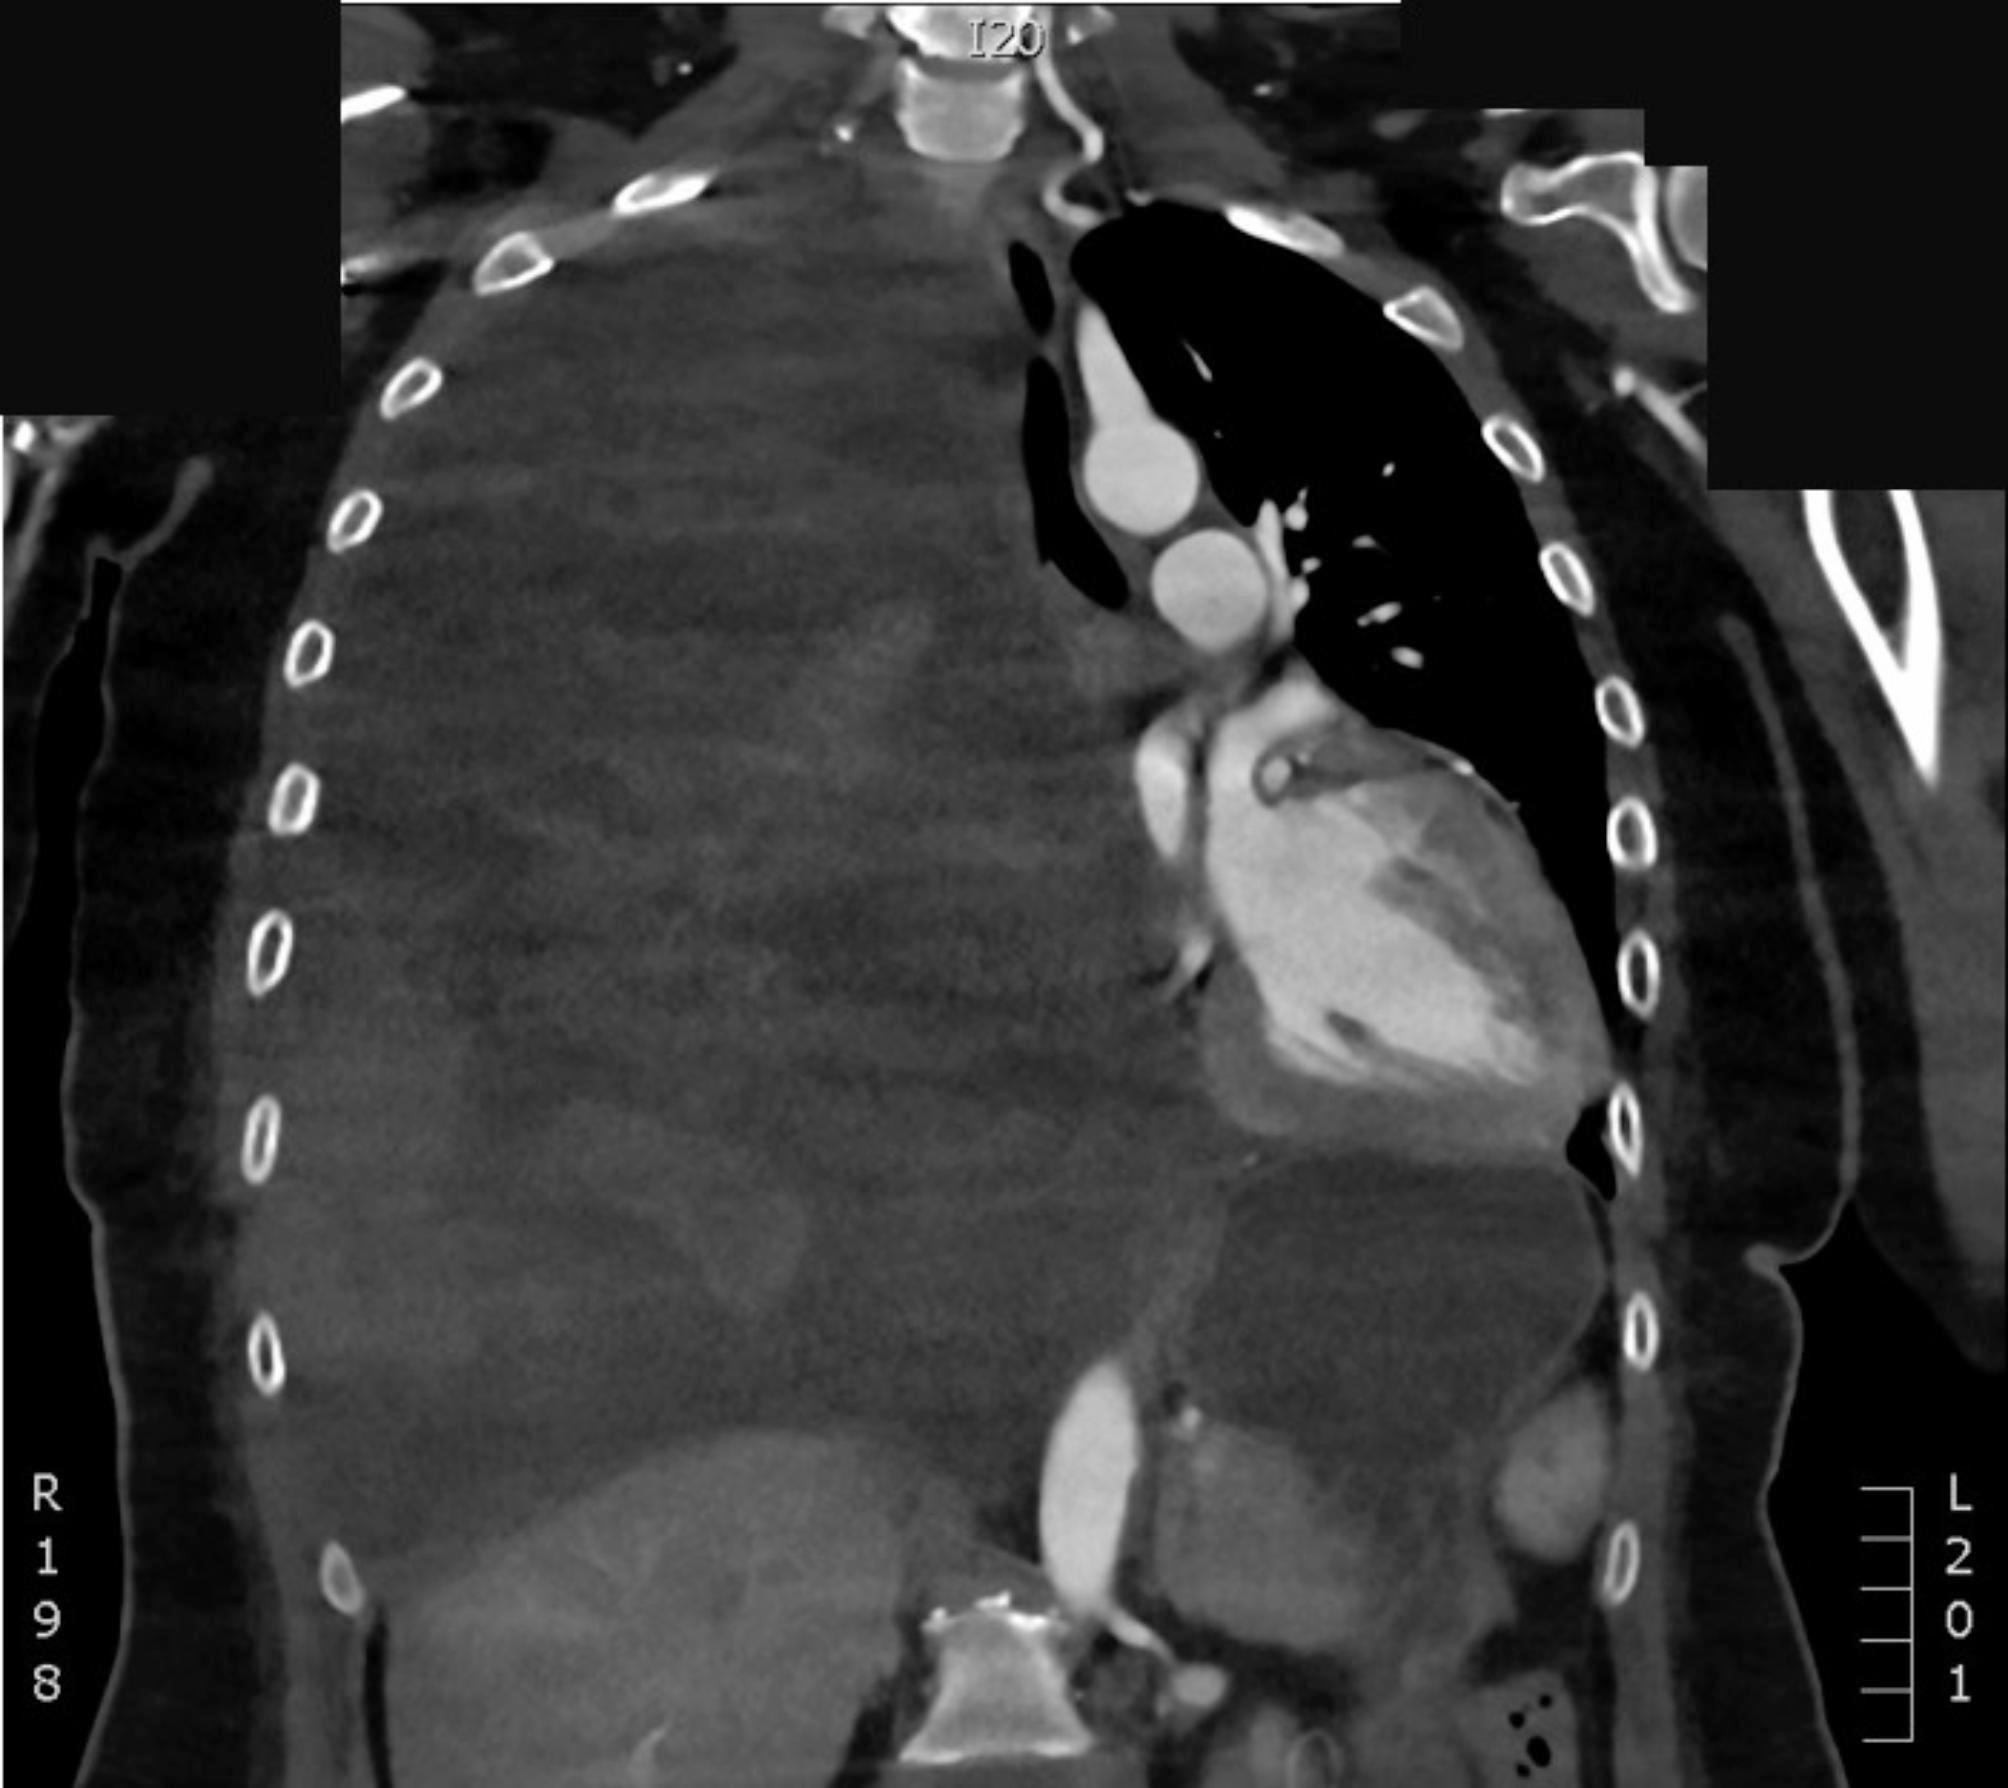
CT angiogram showing a massive right-sided thoracic hematoma that was dislodging the mediastinum. The effusion was compressing the pulmonary arteries and the superior vena cava
